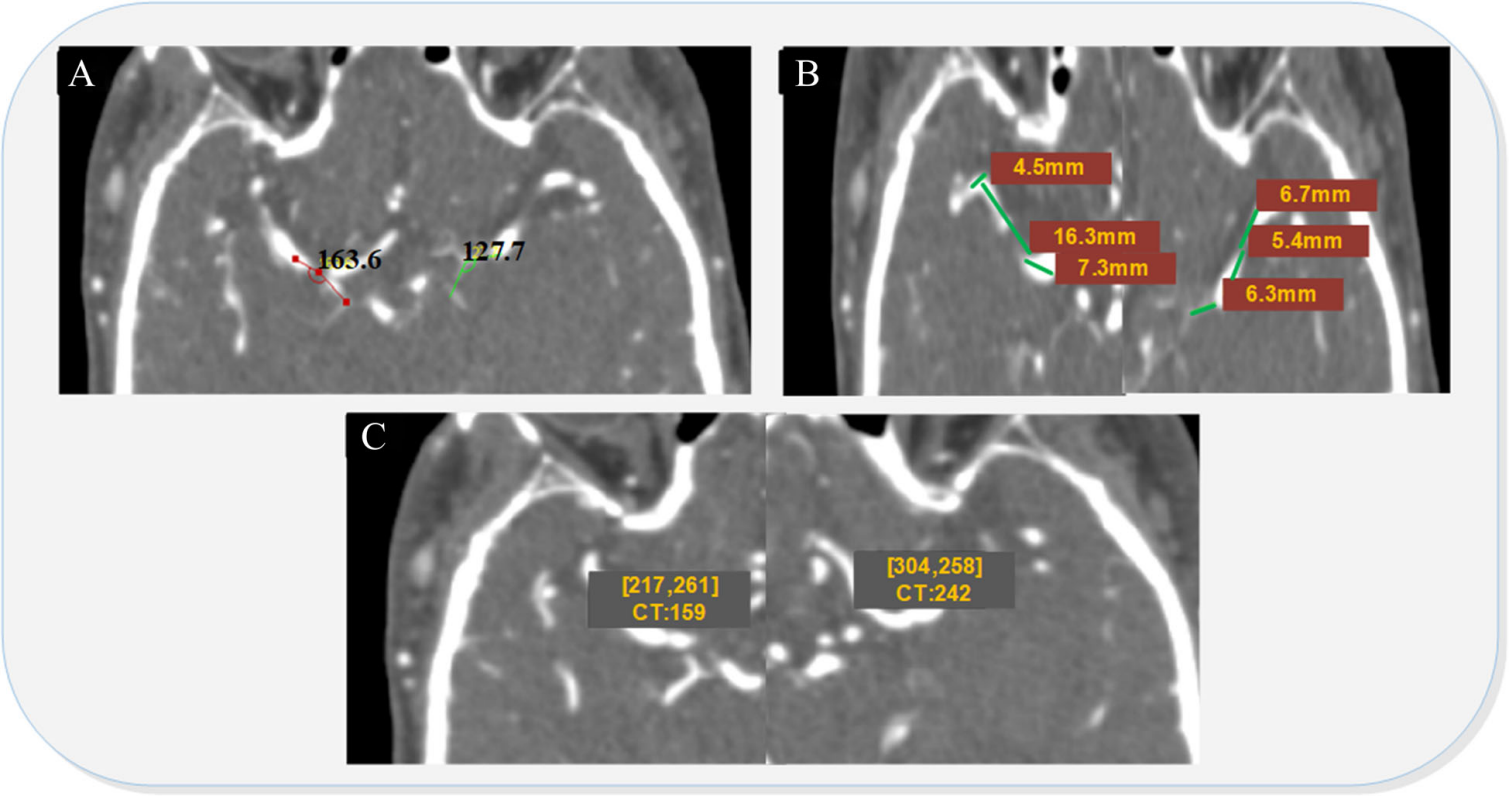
Computed tomography angiography features for the haematoma side and contralateral side of ICH patients
